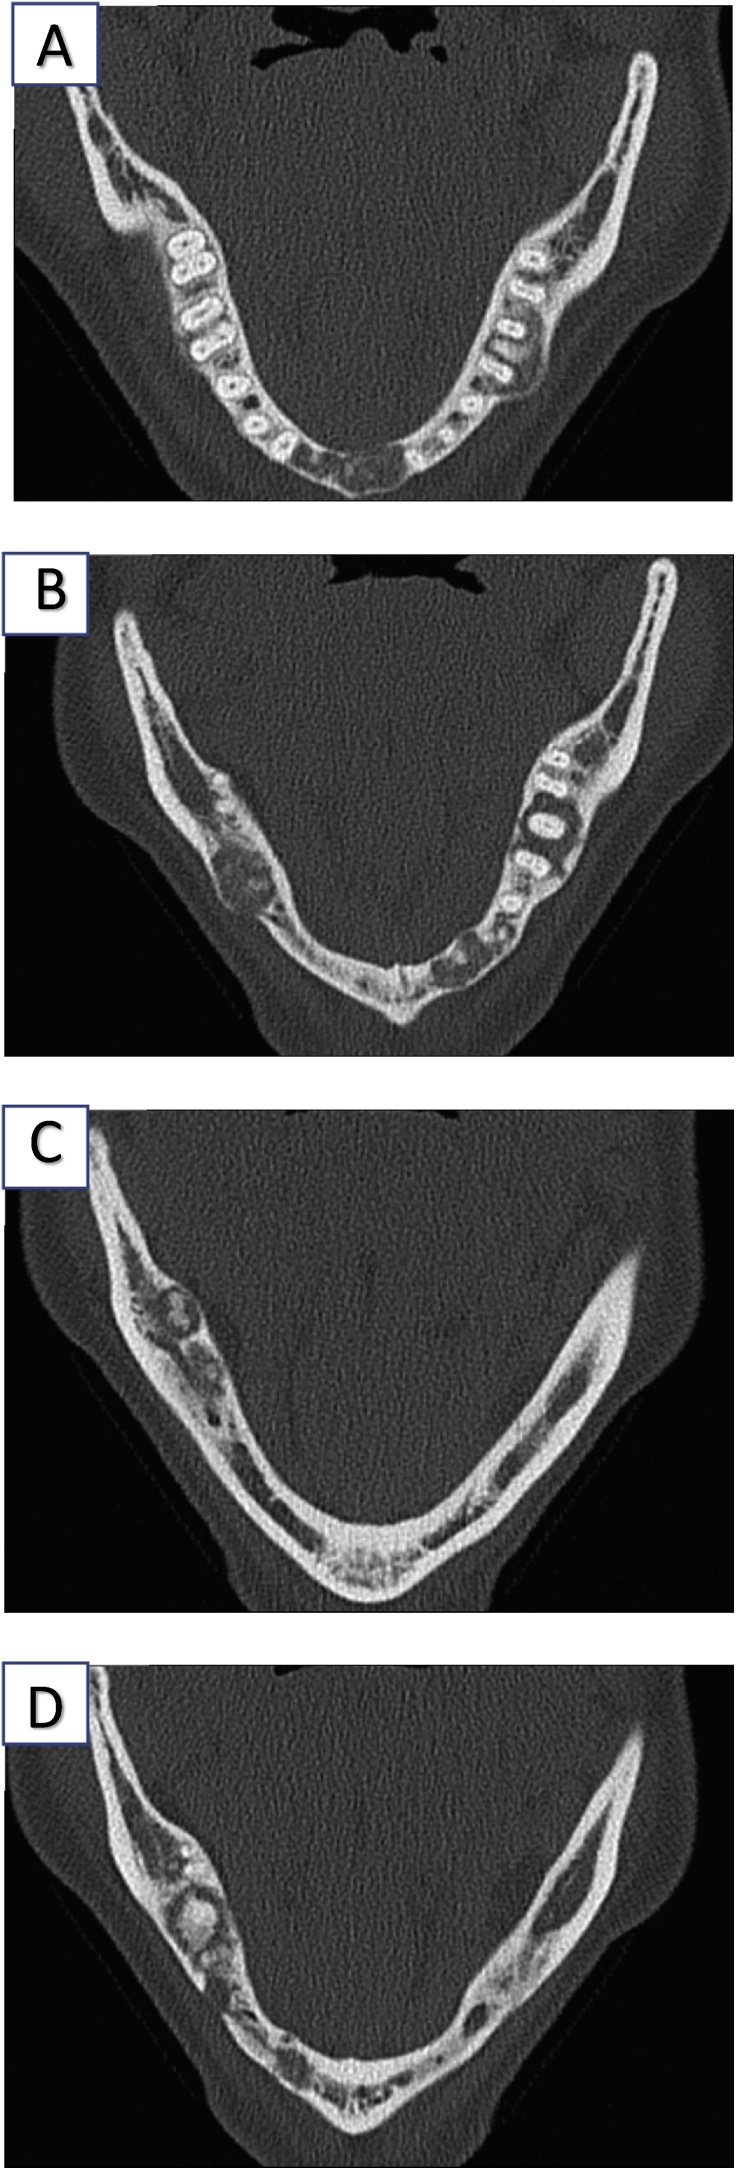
A–D are the computed tomography images, which show the successive layers of the computed tomography in the horizontal cut.
